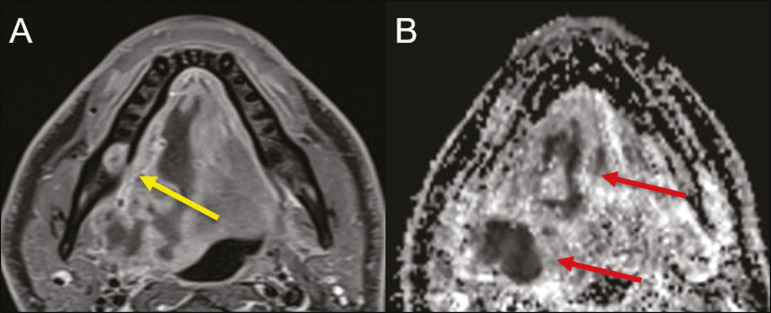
Contrast-enhanced axial T1-weighted MRI sequence of the face (A) and apparent diffusion coefficient map (B) showing extraction (A) of the lower right second molar (yellow arrow) with granulation tissue and signs of alveolar inflammation accompanied by a heterogeneous fluid collection showing restricted diffusion (B), delineating the planes of the floor of the mouth, and extending to the base of the tongue, causing narrowing of the oropharyngeal air column (red arrows).
